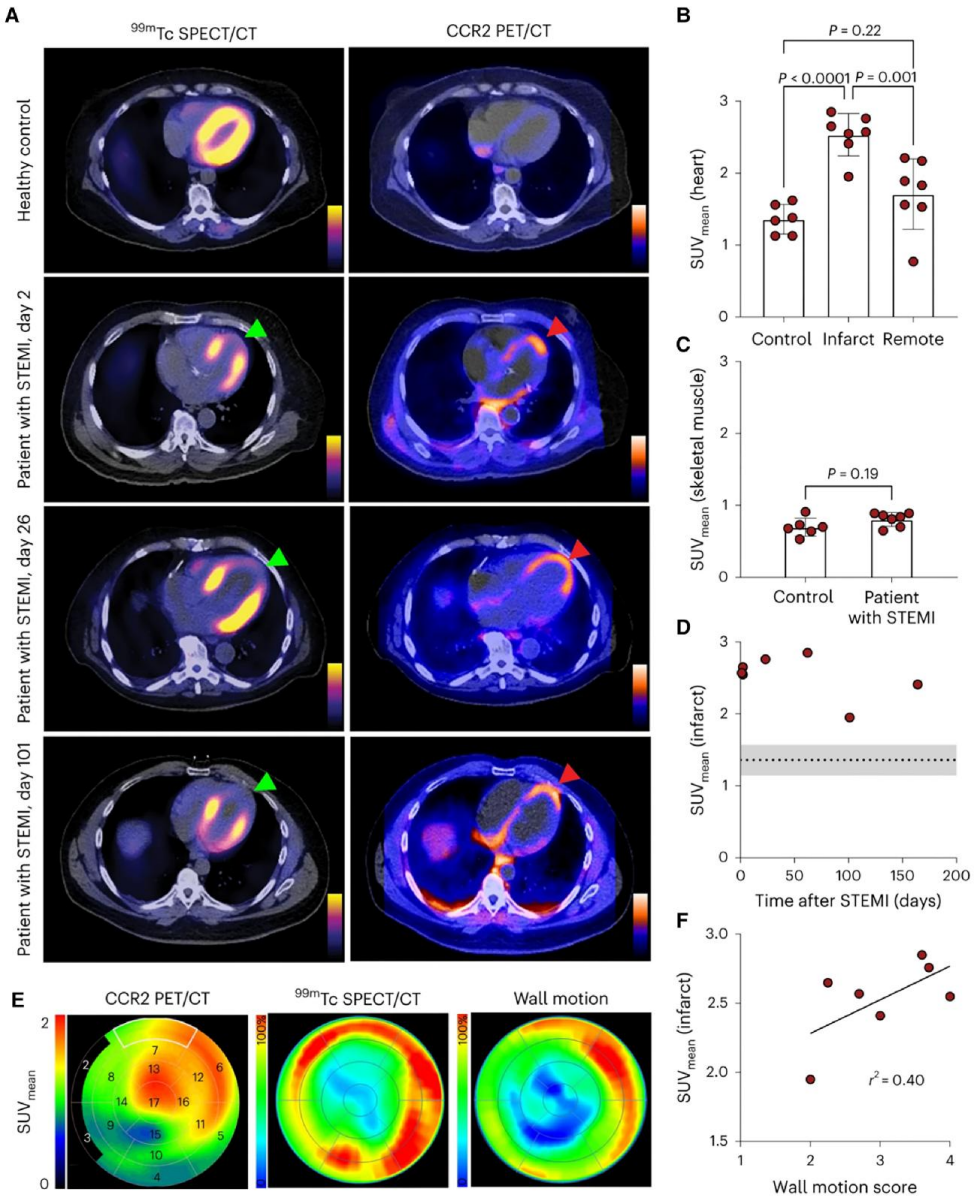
Visualizing myocardial inflammation using ^64^Cu-DOTA-ECL1i PET/CT. *A*) Tc-99m SPECT/CT and ^64^Cu-DOTA-ECL1i PET/CT images from healthy controls and STEMI patients. Regions with increased ^64^Cu-DOTA-ECL1i uptake strongly correlated with infarct areas of reduced perfusion in Tc-99m SPECT/CT (red and green arrowheads). *B, C*) ^64^Cu-DOTA-ECL1i signal intensity (SUV_mean_) was significantly increased in the infarct myocardium of STEMI patients compared to healthy controls. No significant difference in intensity was observed in the skeletal muscle. *D*) ^64^Cu-DOTA-ECL1i signal as a function of time after STEMI (days). Each data point represents an individual STEMI patient, while the line and grey zones represent the mean value of healthy controls with SD. *E*) 17-segment model polar maps representing areas of perfusion and myocardial wall motion from Tc-99m SPECT/CT. *F*) Linear regression analysis showed a significant association between ^64^Cu-DOTA-ECL1i uptake and wall motion score (r^2^ = 0.40, *P* = 0.03). Reproduced from Lavine *et al.*, copyright permission obtained from Springer Nature (CC-BY-NC).^29^
